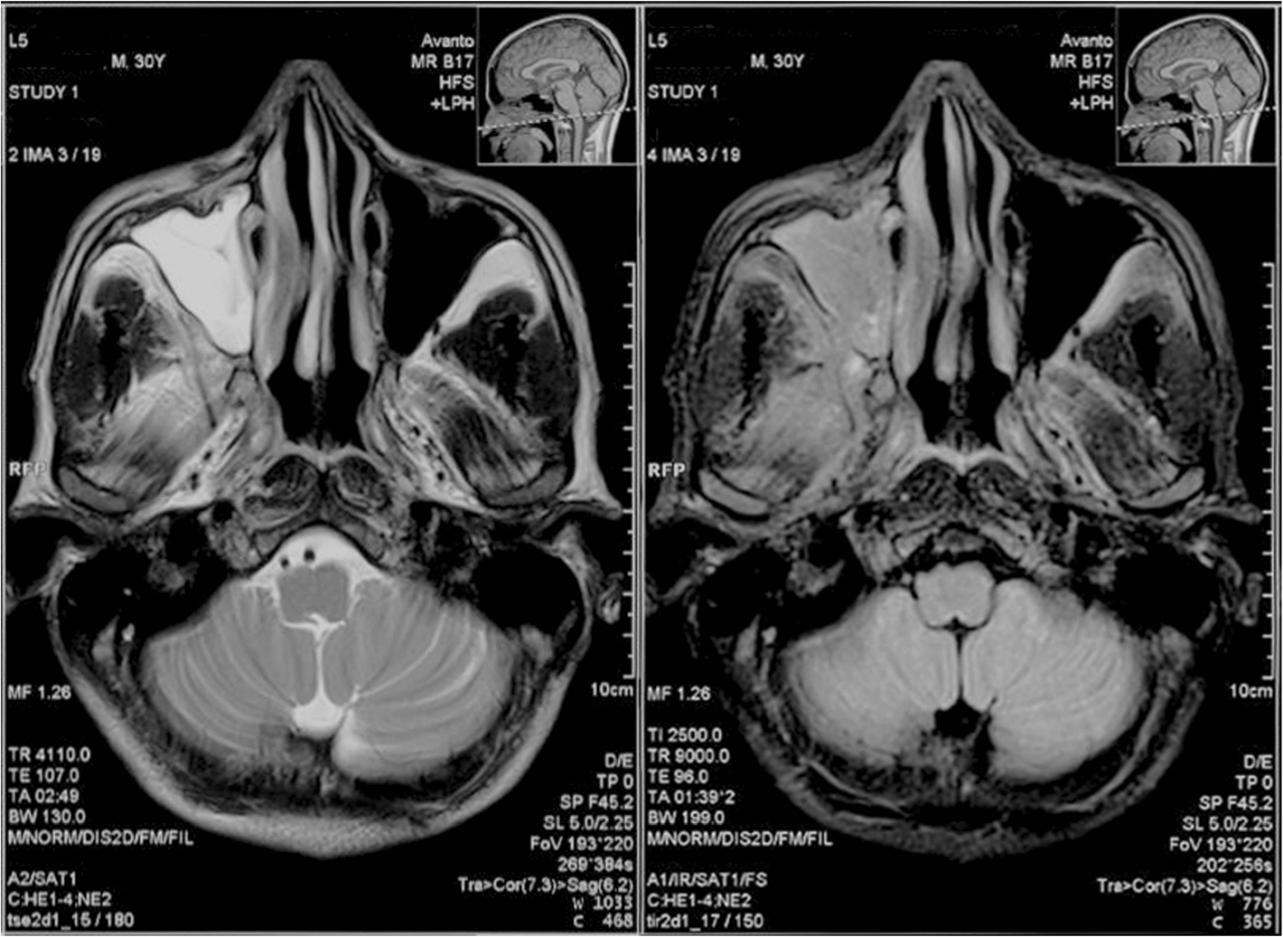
MRI brain. Opacification and mucosal thickening of the right maxillary and ethmoid sinuses. Left – T2 weighted image; right– T1 weighted image
